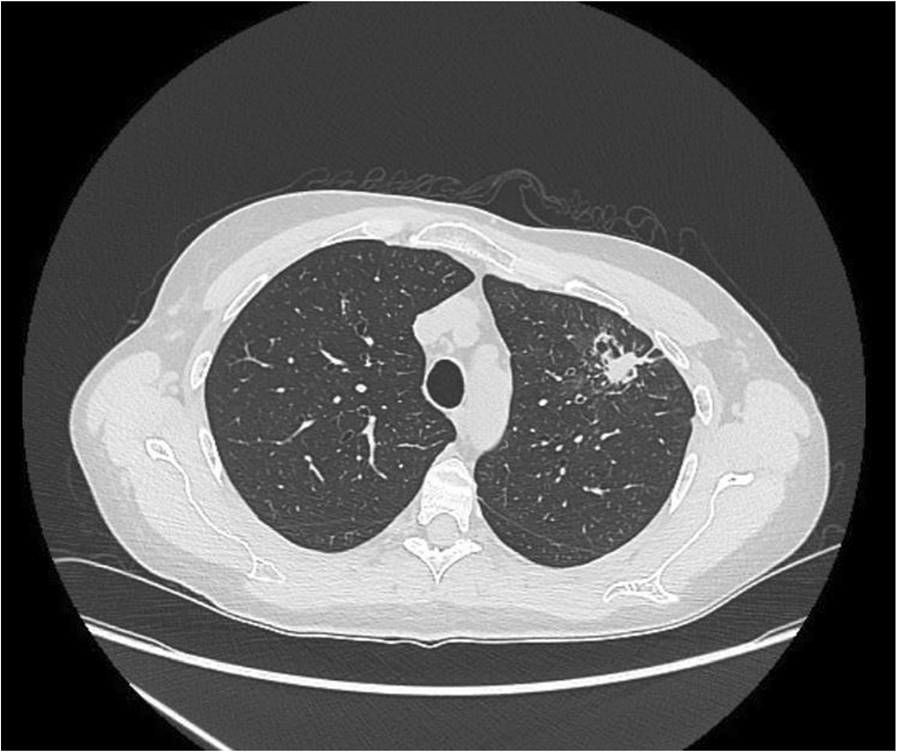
Follow up CT imaging with resolving left upper lobe cavitary lesion after initiation of corticosteroids, azathioprine and anti-IL-5
